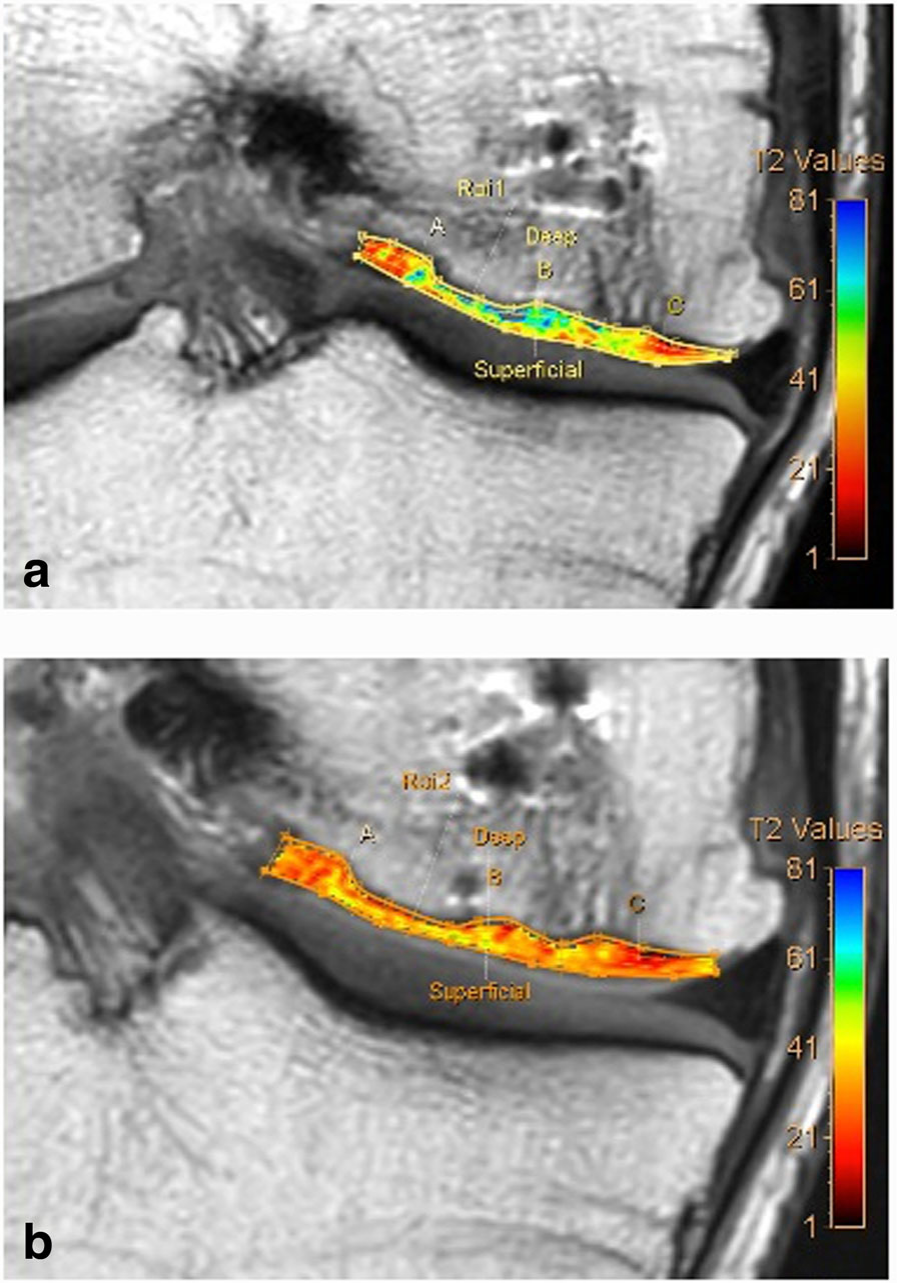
Post-Treatment MRI T2 mapping of the medial compartment of the knee. a 6 month T2 Mapping: Elevated values within the regenerated cartilage indicate immature cartilage or fibrocartilage morphology. b 18 month T2 Mapping: Improved values within both the deep and superficial layers indicate hyaline cartilage. This finding indicates cartilage maturation since previous imaging at 6 months
